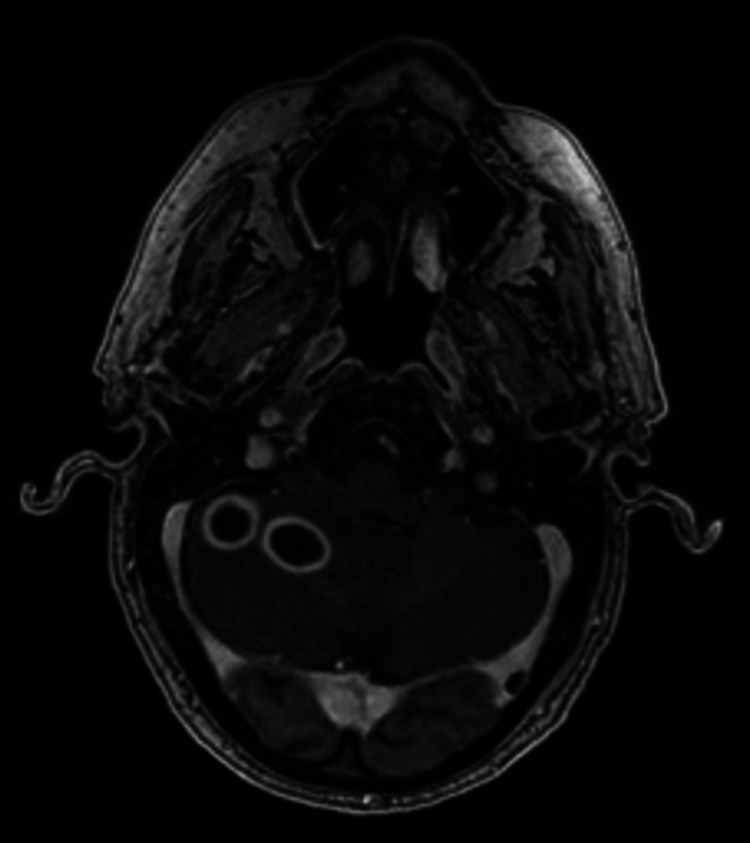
MRI brain with contrast, T1-weighted image showing 2.0 cm and 1.7 cm lesions within the right cerebellar hemisphere
